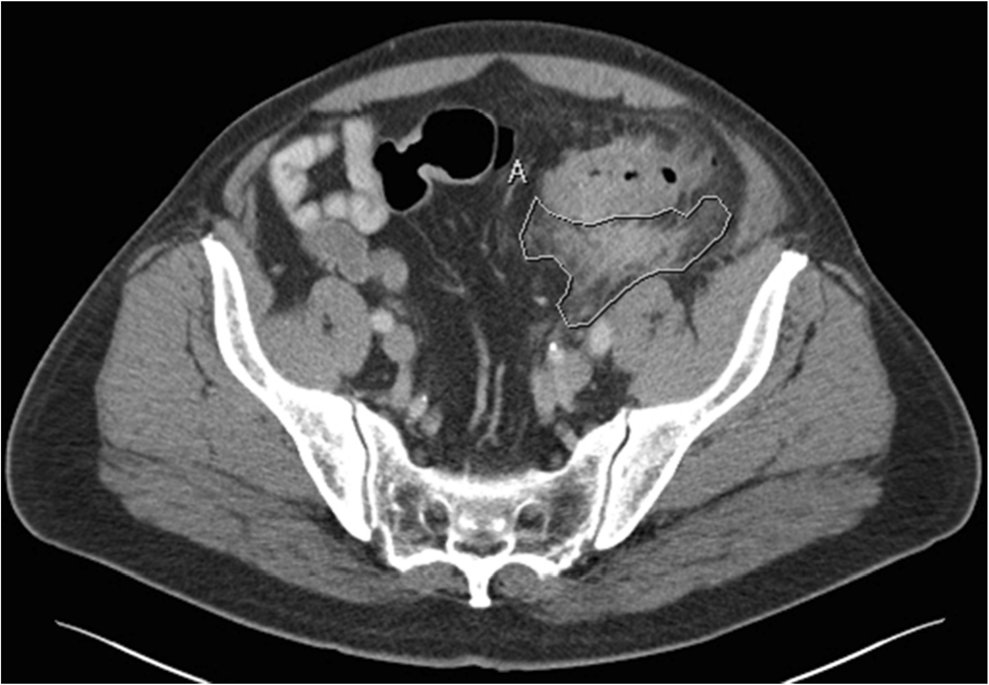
CT slide showing pericolic inflammation secondary to acute diverticulitis. Pericolic inflammation is measured using a mean region of interest (ROI) resulting in an area of inflammation of 23.65 cm^2^ with a mean Hounsfield unit of + 17.40
